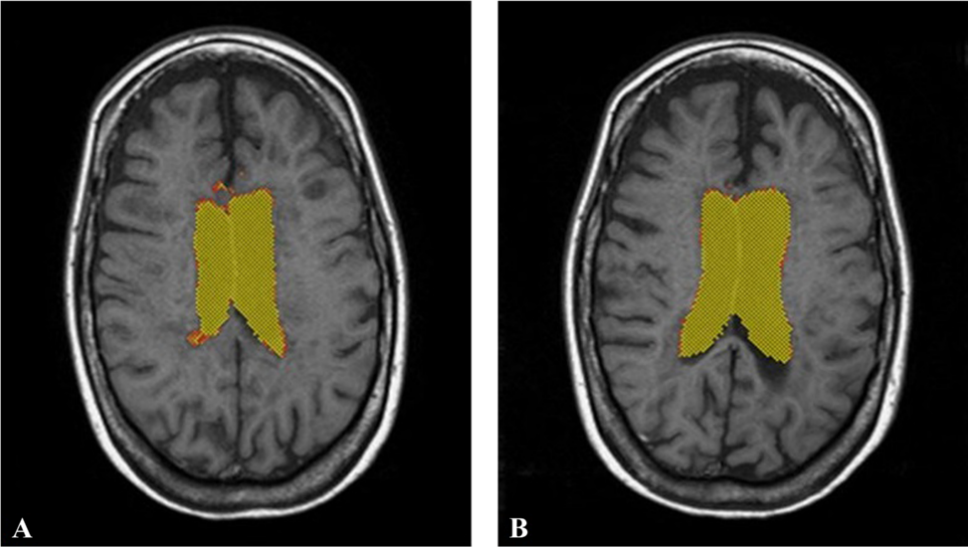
The MRI post-analyisis detected an increase of 17,5 % in cerebro-spinal fluid volume (CSFV) between before (a: CSFV = 94 ml) and after treatment (b: CSFV = 114 ml), as assessed by the Siena tool of FSL software
